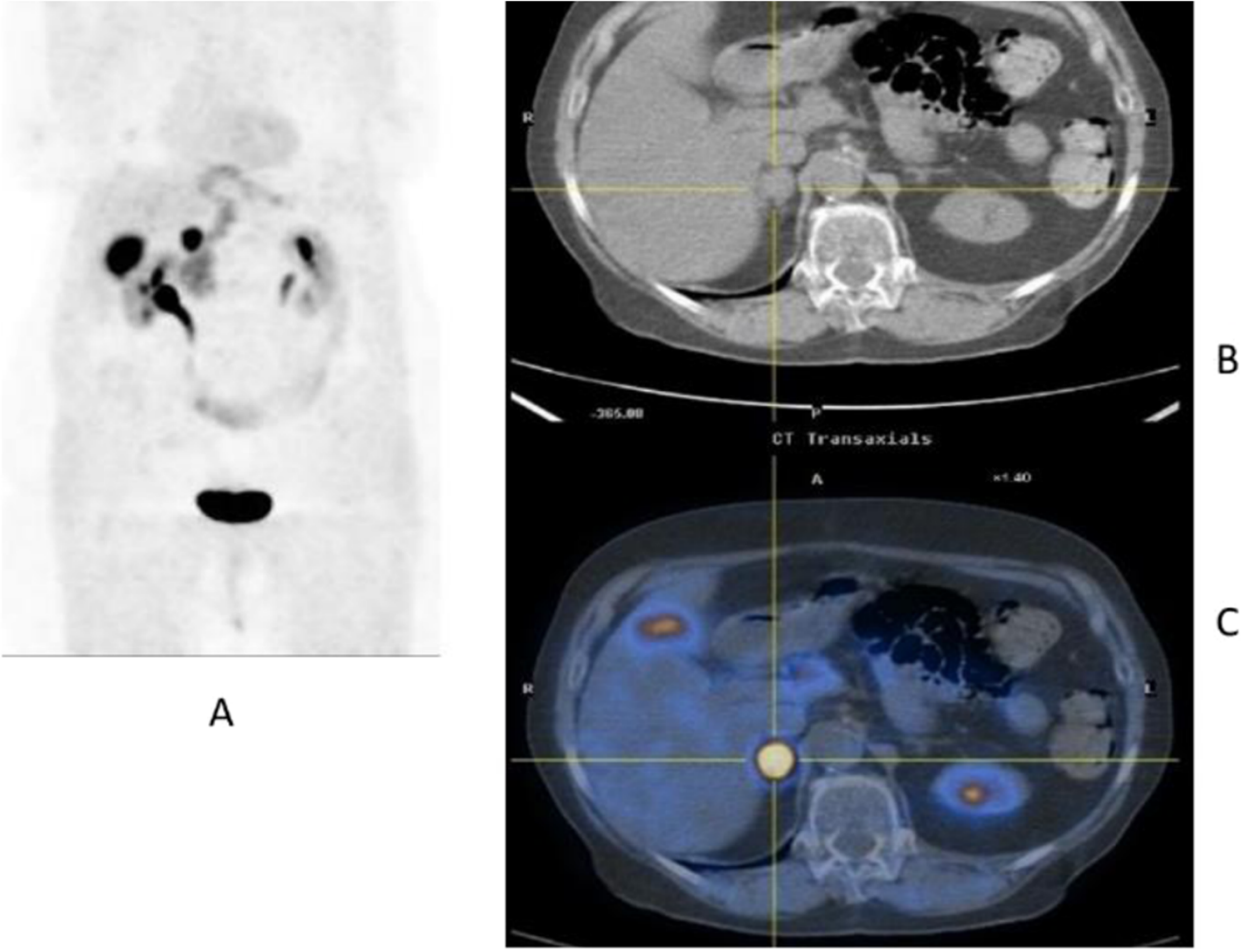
^18^F-FDOPA, pheochromocytoma. *Clinical history*: 45 y.o. man with resistant hypertension. Clinical suspicion of phaeochromocytoma. *PET/CT findings*: intense uptake of the tracer in the right adrenal gland in MIP (a), which is enlarged on CT (b) and fused images (c). Surgery confirmed presence of phaeochromocytoma
